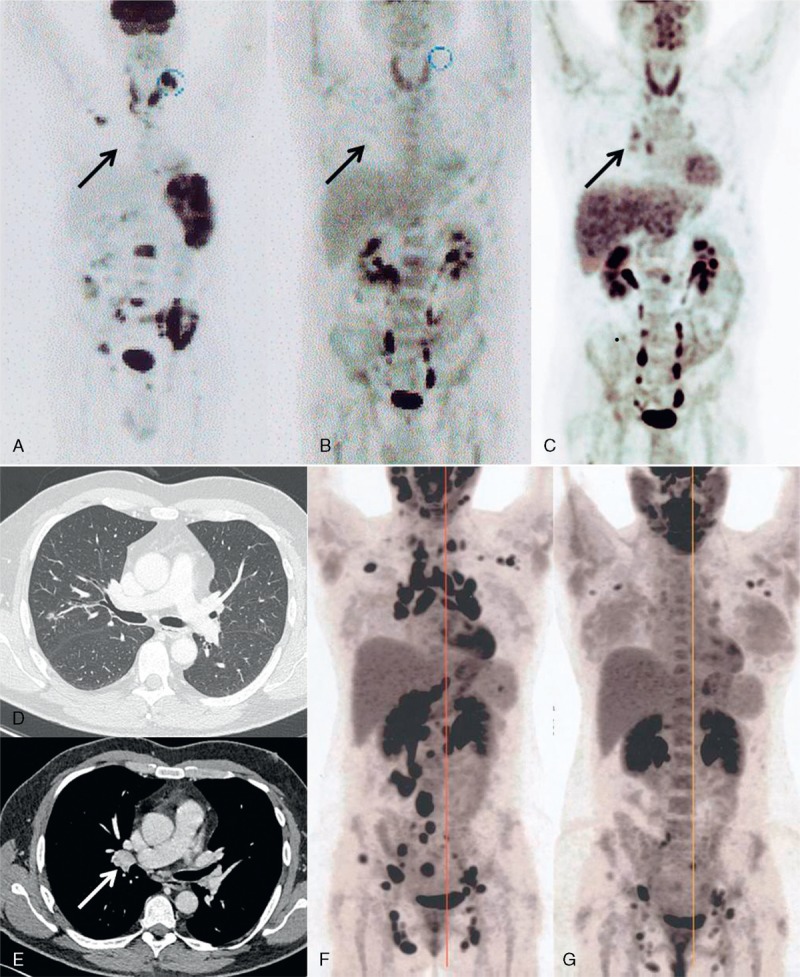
Radiological evolution of lymphoma and sarcoidosis. Positron Emission Tomography/Computerized Tomography (^18^FDG-PET/CT) of patient 1 at lymphoma diagnosis (A), lymphoma remission after diagnostic splenectomy and chemotherapy (B) and at sarcoidosis diagnosis 2 months later (C). Black arrow indicates the occurrence of moderately hypermetabolic (SUVmax = 3.5) hilar lymphadenopathy at sarcoidosis onset. CT scan at sarcoidosis diagnosis of patient 1 (D and E) shows micronodules in the middle lobe (D) and a 19 mm hilar enlarged lymph node (white arrow, panel E). Panel F and G are ^18^FDG-PET/CT evaluations of patient 2 at sarcoidosis diagnosis (F) and at last follow-up, 6 months after introduction of steroid treatment (panel G) showing a very good PR. PR = partial response, SUV = standardized uptake value.
